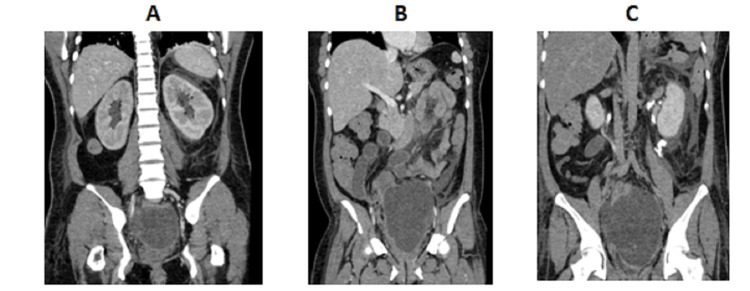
Coronal CT scan with IV contrast (following abnormal results of renal function tests) (A,B) Images showing bilateral renal calyceal dilatation, left perirenal fat stranding, and large hypodense pelvic collection. (C) Delayed images showing contrast extravasation from the left calyceal system and pelvic collection. Following abnormal results on renal function tests, a CT scan was performed to investigate the cause of the altered renal function, which ultimately led to the diagnosis of bilateral ureteric injuries and a urinoma. CT, computed tomography
